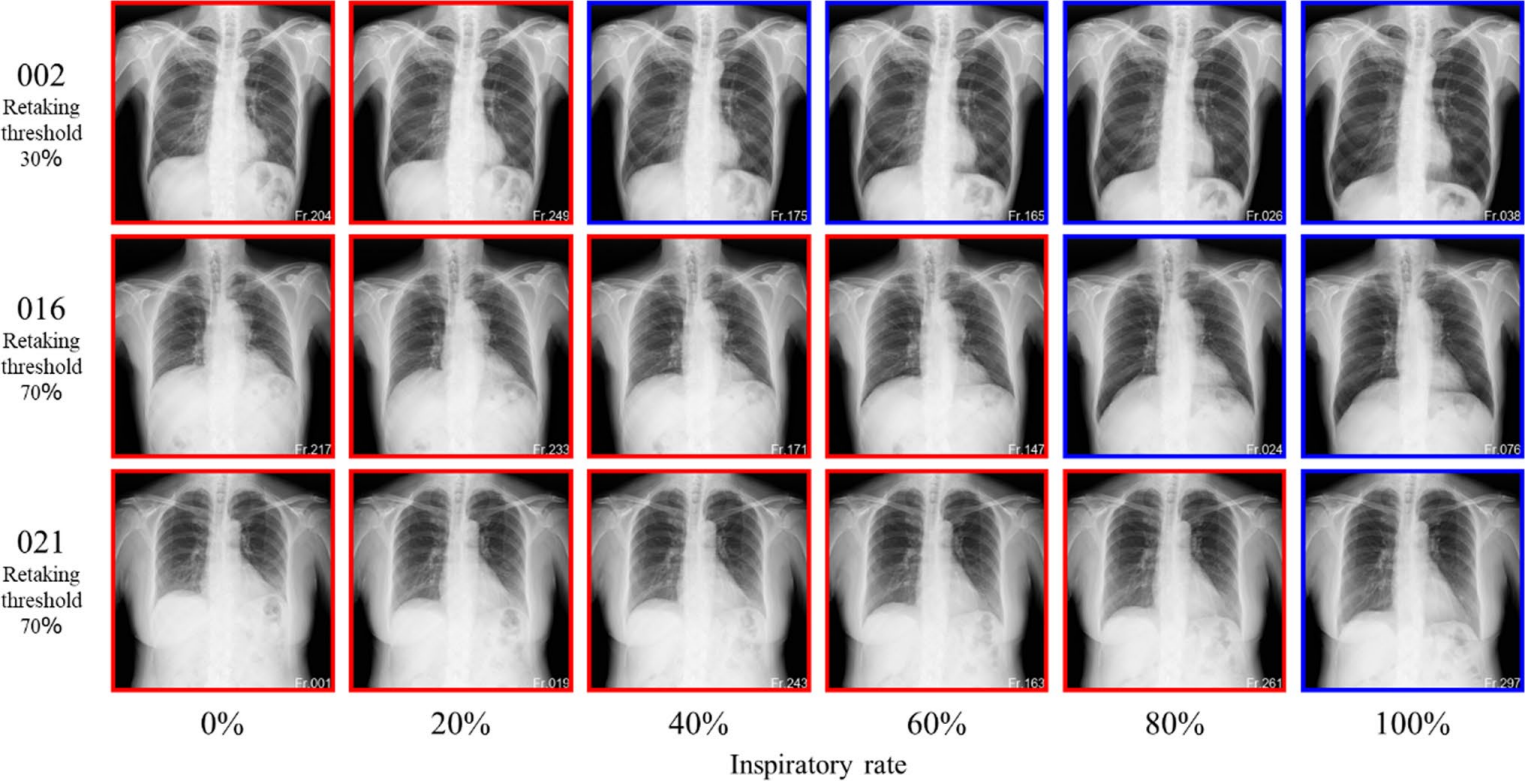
Assessment results for representative inspiratory rate images. The color of the outer border of the image indicates whether the radiograph was assessed as requiring retaking. The red line indicates that the image was assessed as “Retake,” and the blue line indicates that the image was assessed as “Complete.”
